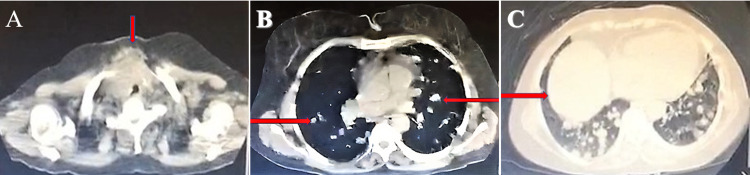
Patient's spiral chest CT scan showed (A) two nodules in the isthmus of the thyroid gland (as shown by the red arrow), (B) multiple cotton wool nodules in the parenchyma of the bilateral lungs (as shown by the red arrows), and (C) a hypodense lobular mass at the thoracic inlet extending into the anterior mediastinum (as shown by the red arrow), consistent with metastatic disease.
